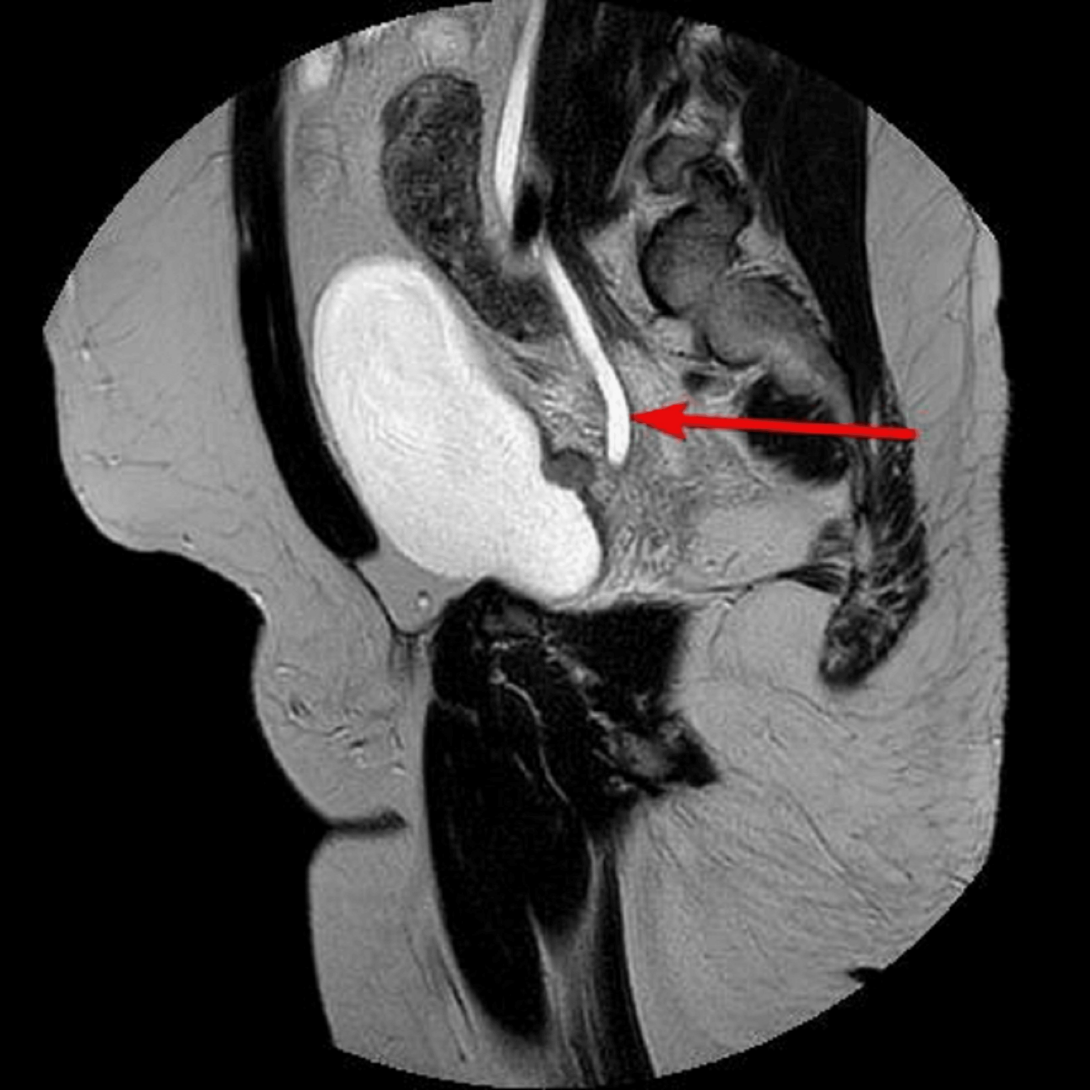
Right hydroureter appreciated on MRI.
